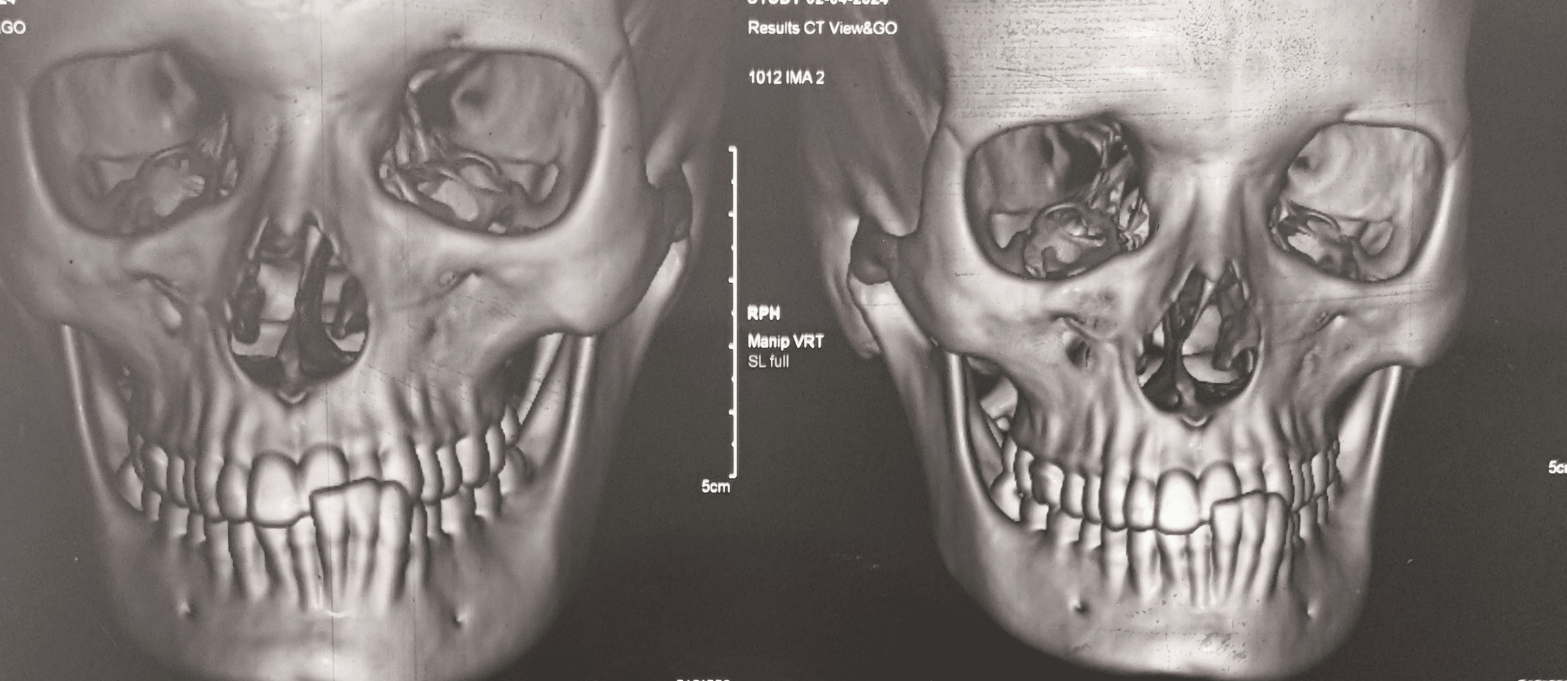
Computed tomography (CT) of the craniofacial skeleton CT imaging demonstrating atrophy of the right zygomatic, maxillary, and mandibular bones, with inferior positioning of the right orbital floor compared to the left.
